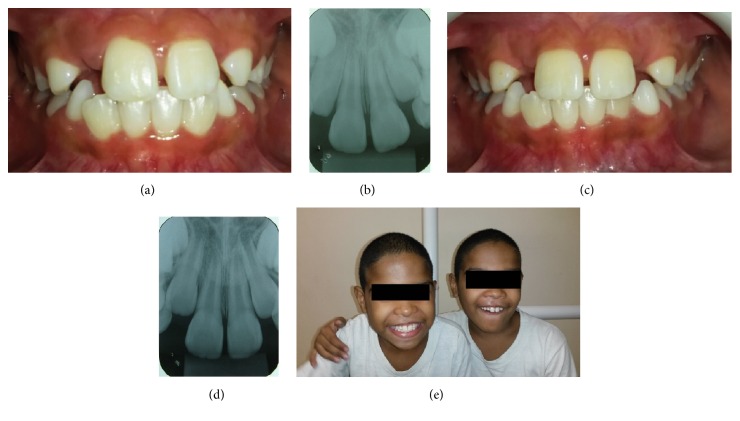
Six months later, after treatment. (Case  1: (a), (b); Case  2: (c), (d)) Central incisors were in normal position and there was no relapse. Periapical radiographs of upper central incisor teeth. There was no pathological condition ((e): twin boys smiling).
